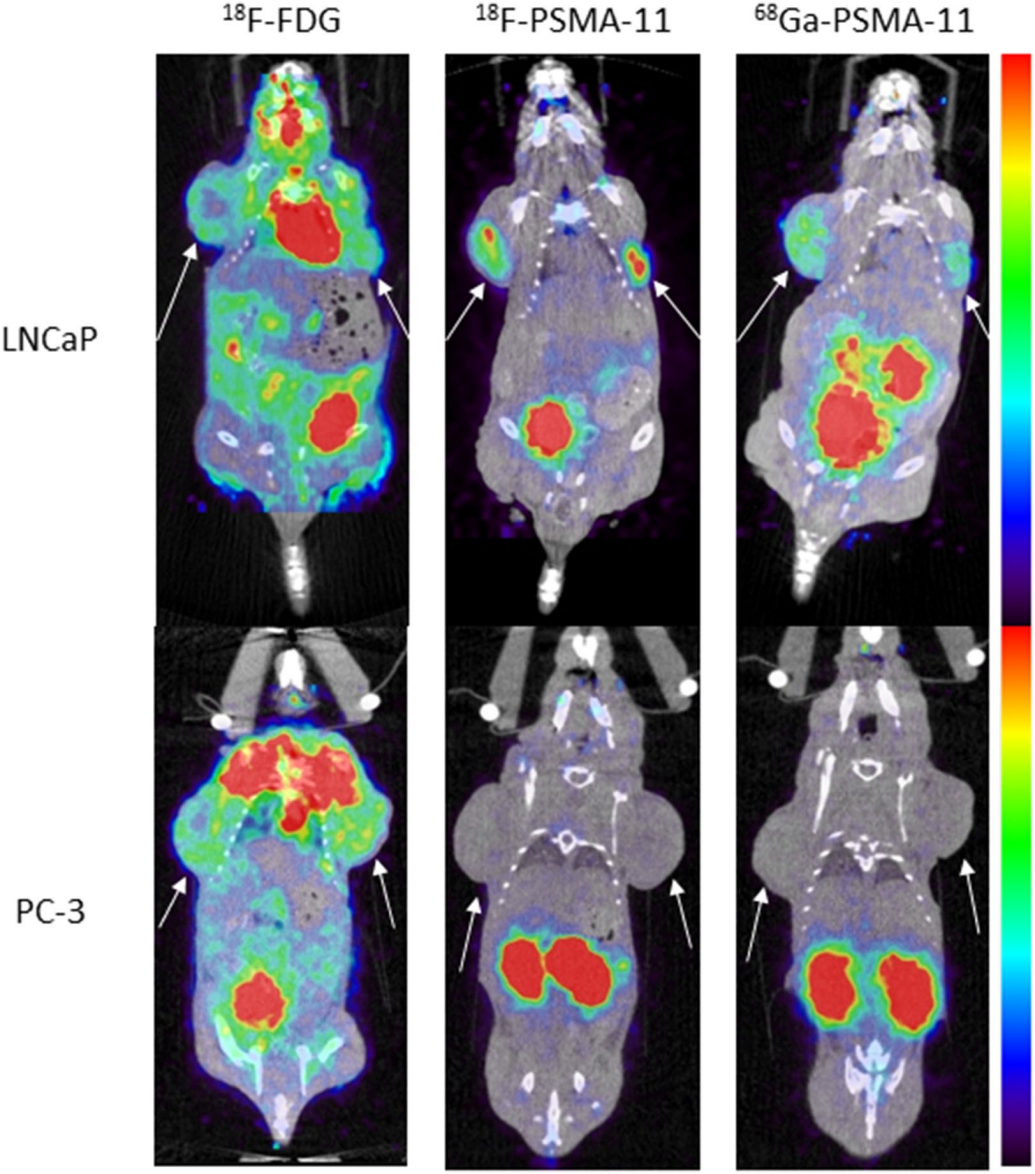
Comparison of ^18^F-FDG, ^18^F-PSMA-11 and ^68^Ga-PSMA-11 uptake in PSMA-positive (LNCaP) and PSMA-negative (PC-3) tumors (indicated by white arrows) 1 h p.i. Colormaps were adapted in order to optimally visualize the tumor.
